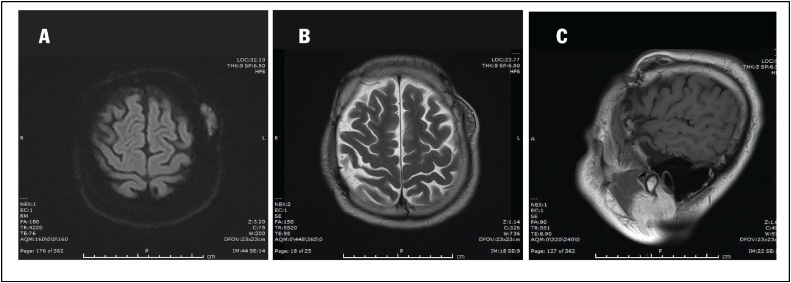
A. Pre-contrast Sagittal T1-weighted sequence demonstrated a well-circumscribed mass separated from the calvarium with isointense to grey matter. B. Pre-contrast axial T1-weighted sequence demonstrated a well-circumscribed mass in the left frontoparietal scalp. C. Pre-contrast axial T2-weighted sequence presented an isointense to grey matter mass.
